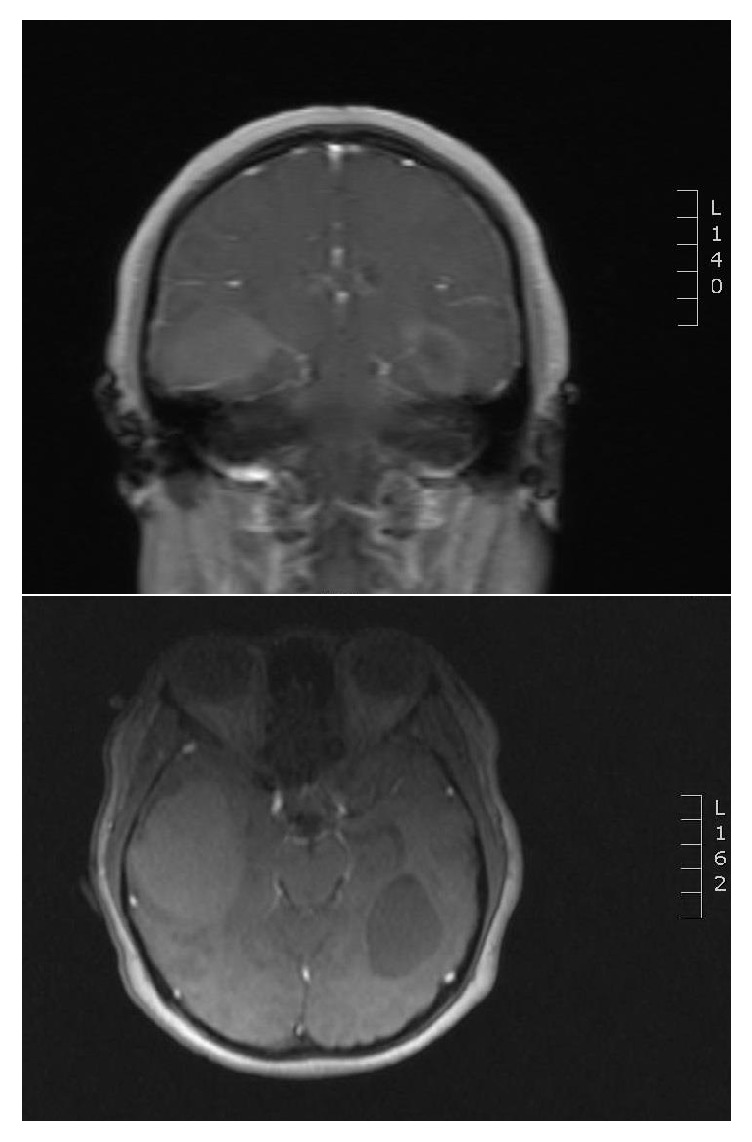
MRI of the brain was done which revealed 4 masses with 2 dominant mass lesions (6.0 and 4.5 cm) having irregular lobulations in the bilateral temporal lobes consistent with metastatic disease.
